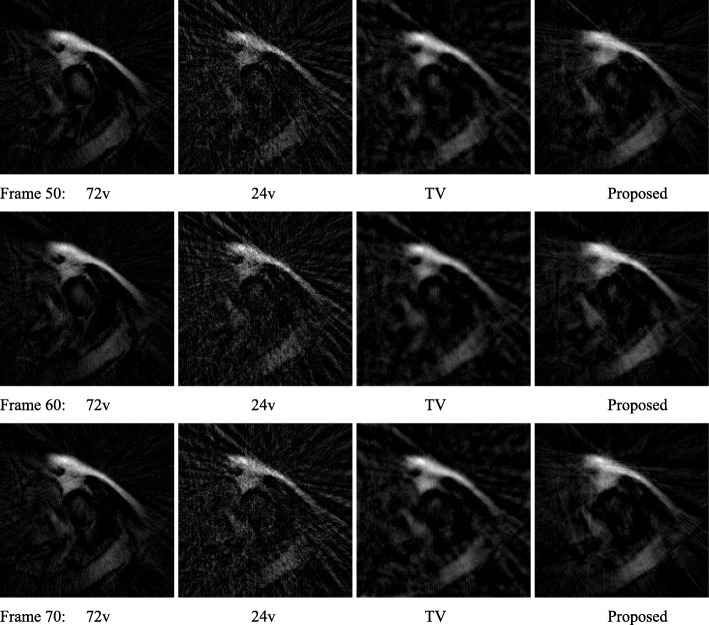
(Frame 50 ~ 70). MRI patient image reconstructions by using (1st column) 72-view FBP, (2nd column) 24-view FBP, (3rd column) 24-view iterative TV, and (4th column) 24-view proposed method. The 1st column is treated as the gold standard for other columns to compare with. The three rows of images correspond to time frames of 50, 60 and 70, respectively
